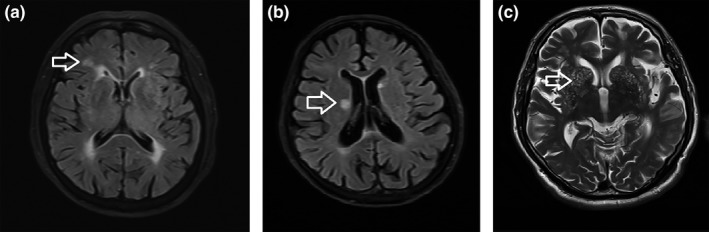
Representative MRI images of white matter abnormalities (a), lacune (b), and enlarged VRS (c) in different regions of the brain in one of the OSAHS patients
